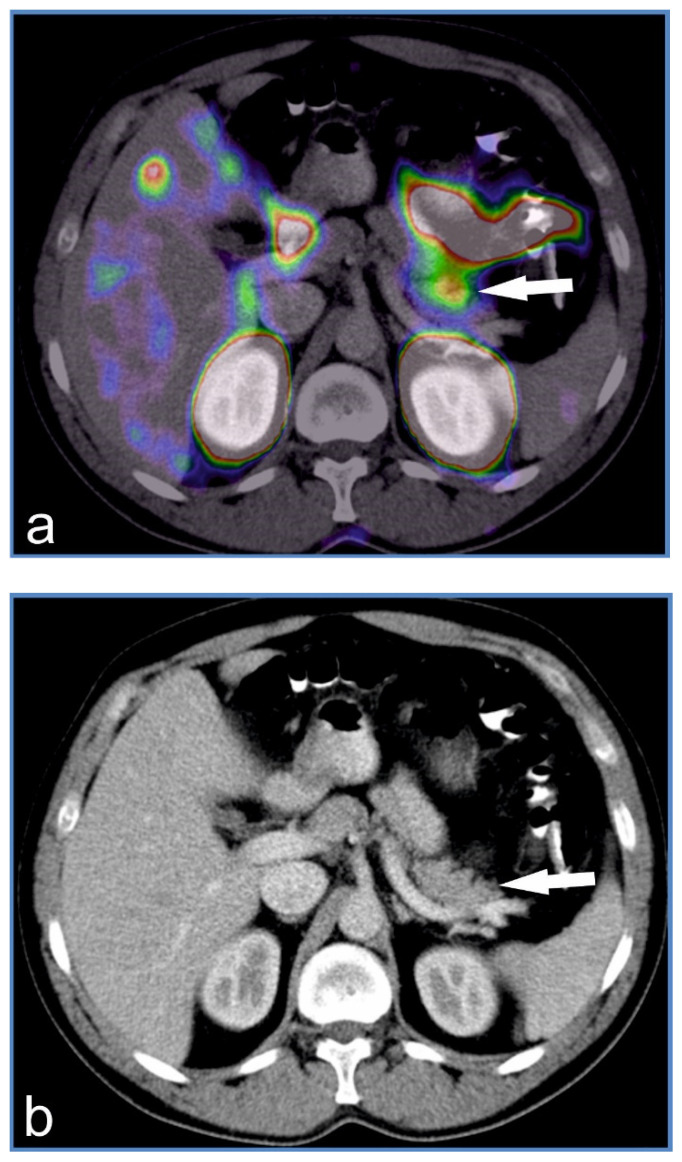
Diffused uptake of tracer found in the body/tail of the pancreas observed via 99mTc-GLP-1 scintigraphy (a) and transverse abdominal computed tomography imaging of the pancreas (b) in patient I.1 [21].
